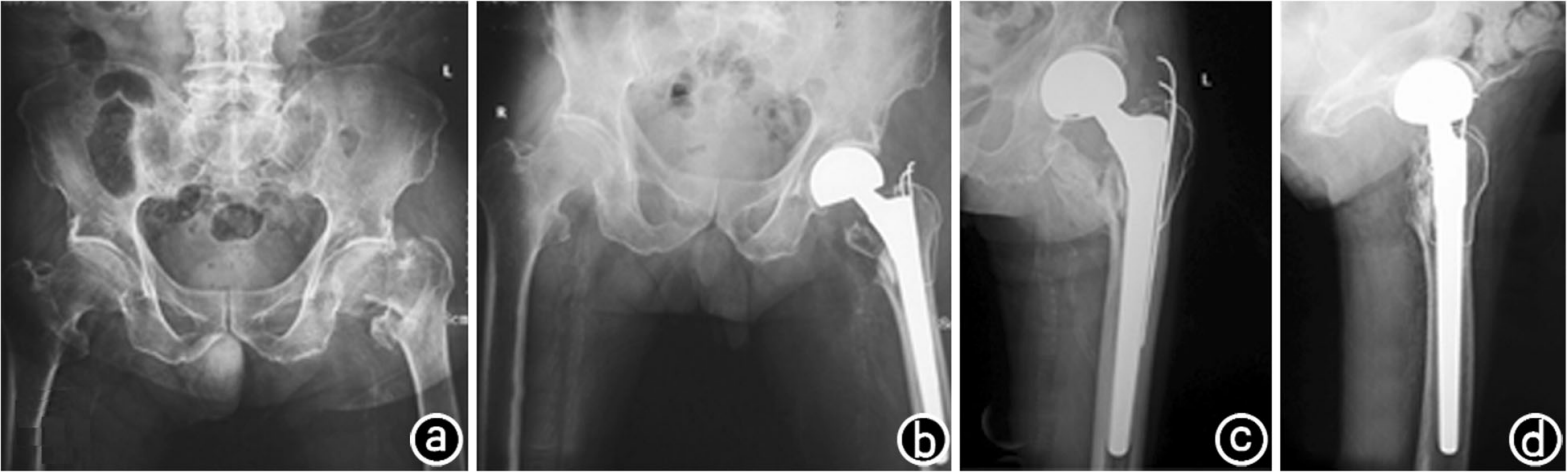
The patient was a 94-year-old male whofell from a height accidentally on November 12, 2011, causing left hip pain and limited activity. a. X-ray examination showed a left intertrochanteric comminuted fracture; b. Physical examination: mild adduction rotation deformity ofhis left hip; the left limb was approximately2cm shorter than the right limb. On November 15, 2011, he underwent left cementless bipolar hemiarthroplasty (CPH) under spinal anes thesia and Kirschner wire bunched fixation. Postoperative X-ray examination showed good positioning of the fracture and satisfactory positioning of the femoral head prosthesis and wire fixation; c, d. X-ray examination 2 years after the operation showed the left femoral head prosthesis in a good position, with no loosening or dislocation
